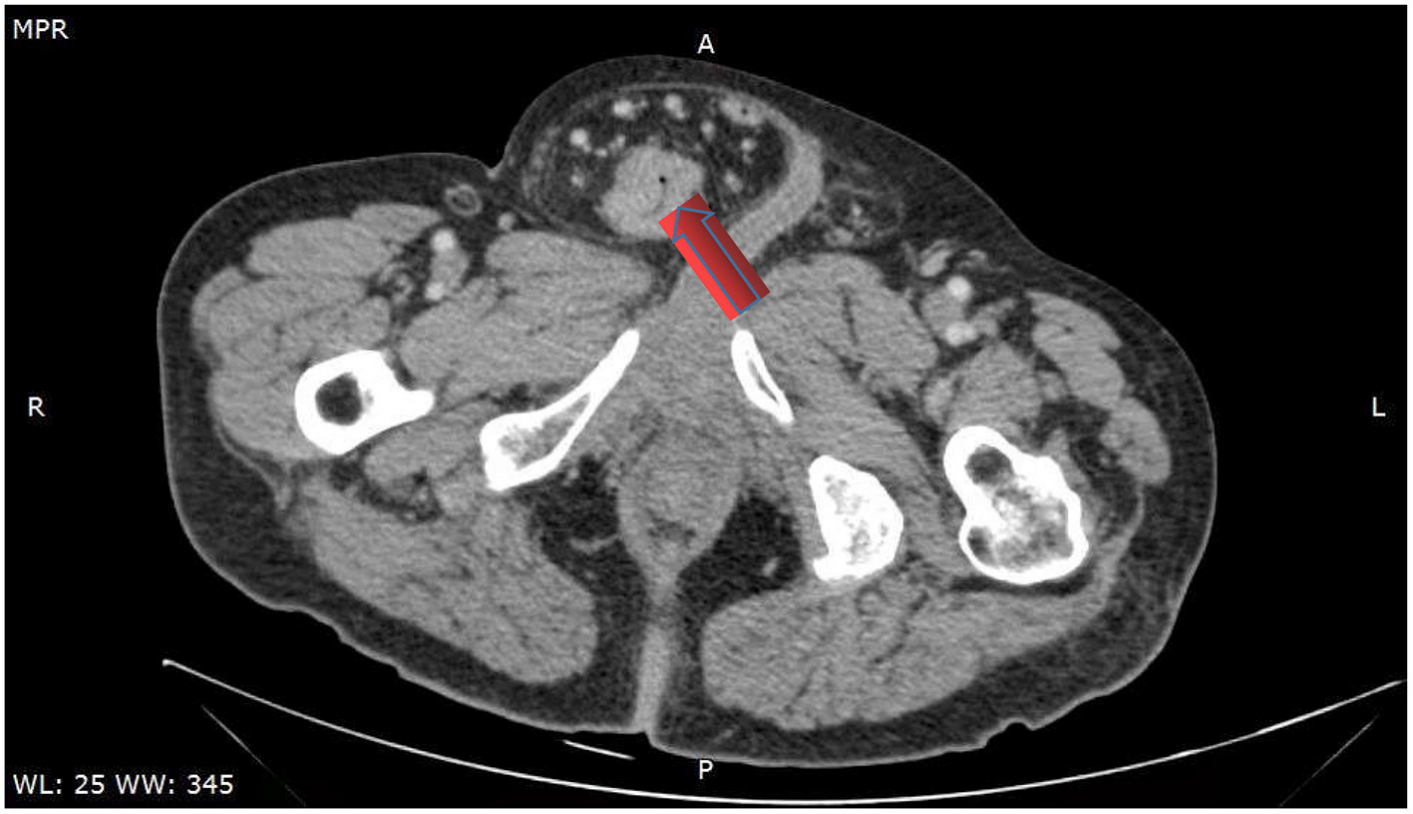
Computed tomography scan showing localized wall thickness of the sigmoid colon in the right groin.
